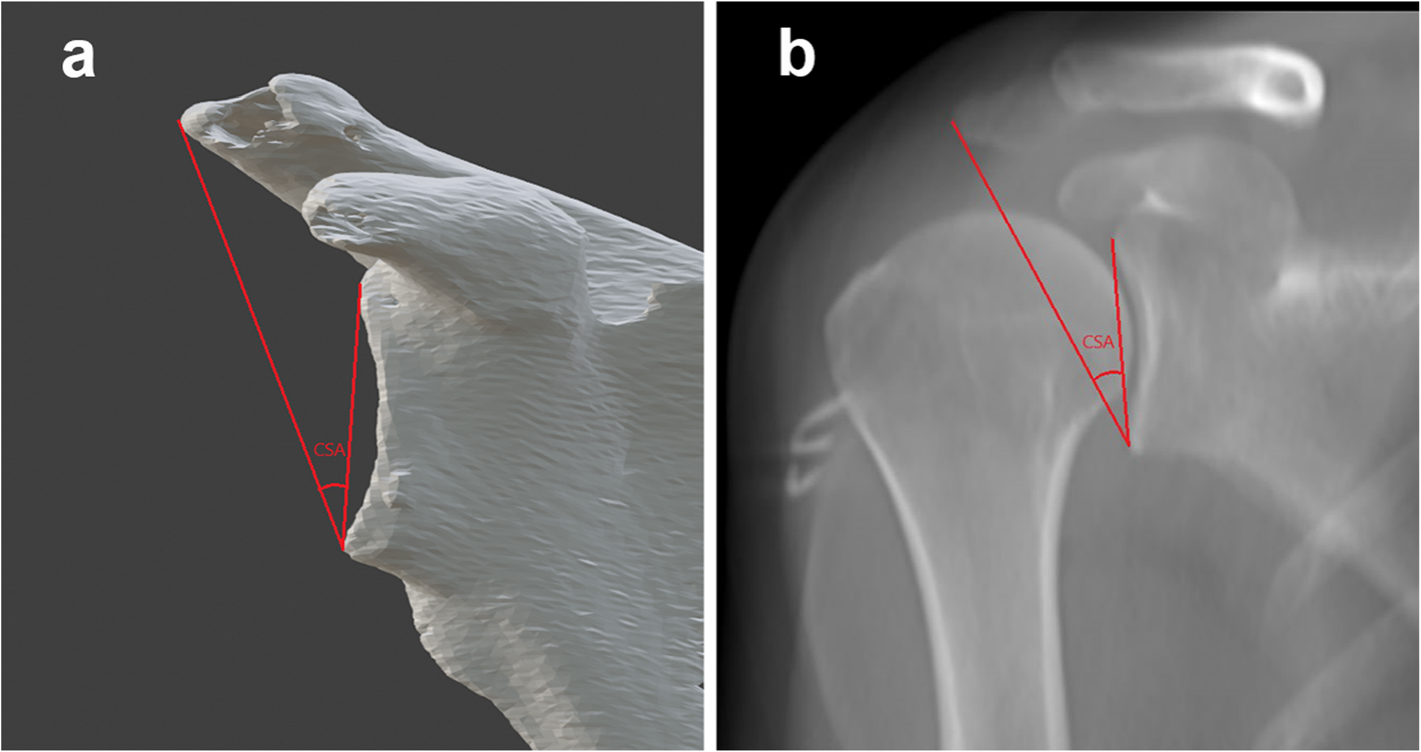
The measurement of the critical shoulder angle (CSA) on 3D scapula (a) and X-ray (b)
